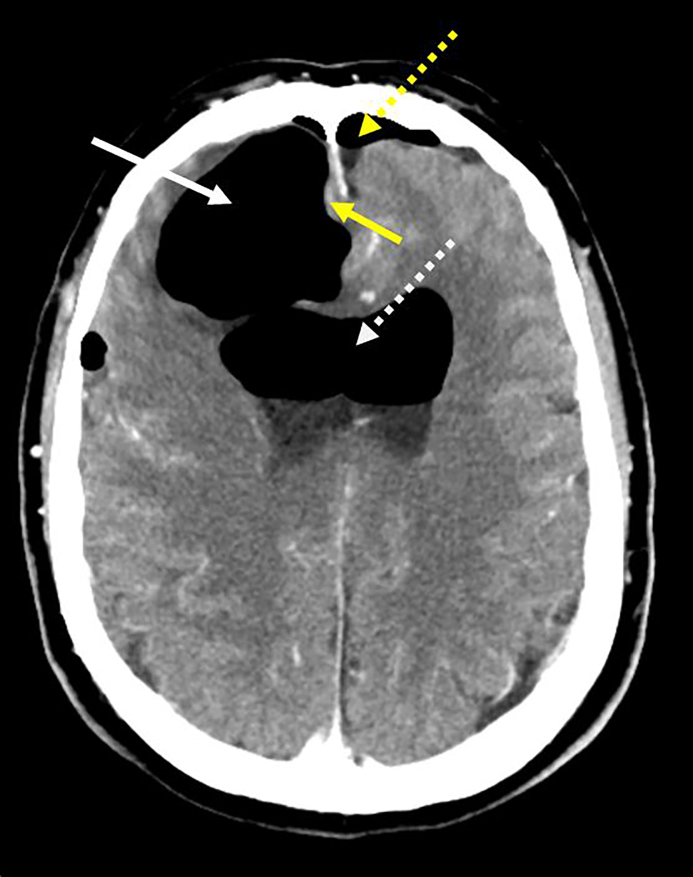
Computed tomography (axial view) of the brain demonstrating extensive, right greater than left, frontal pneumocephalus (solid white arrow), subarachnoid (yellow dotted arrow) and bilateral frontal ventricular horn gas (white dotted arrow) without midline shift. The CT also demonstrates minimal subdural blood (yellow arrow) along the inferior-anterior margin of the interhemispheric fissure adjacent to the gyrus rectus.
